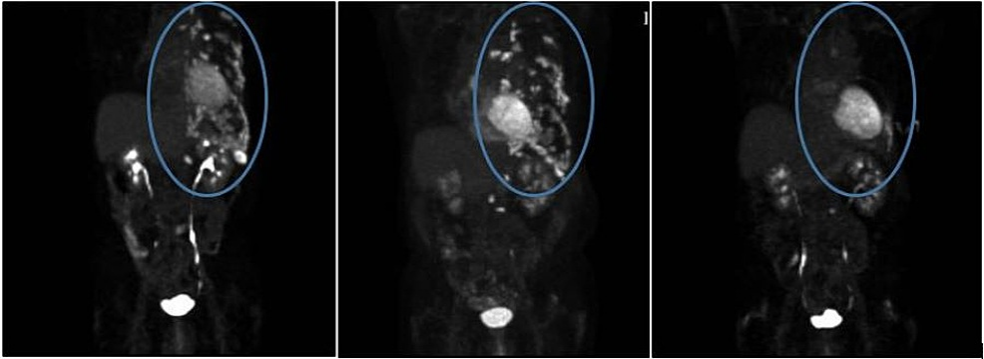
Image on left showing PET/CT at diagnosis, middle image with PET at time of progression, and right image demonstrating PET with partial response.
